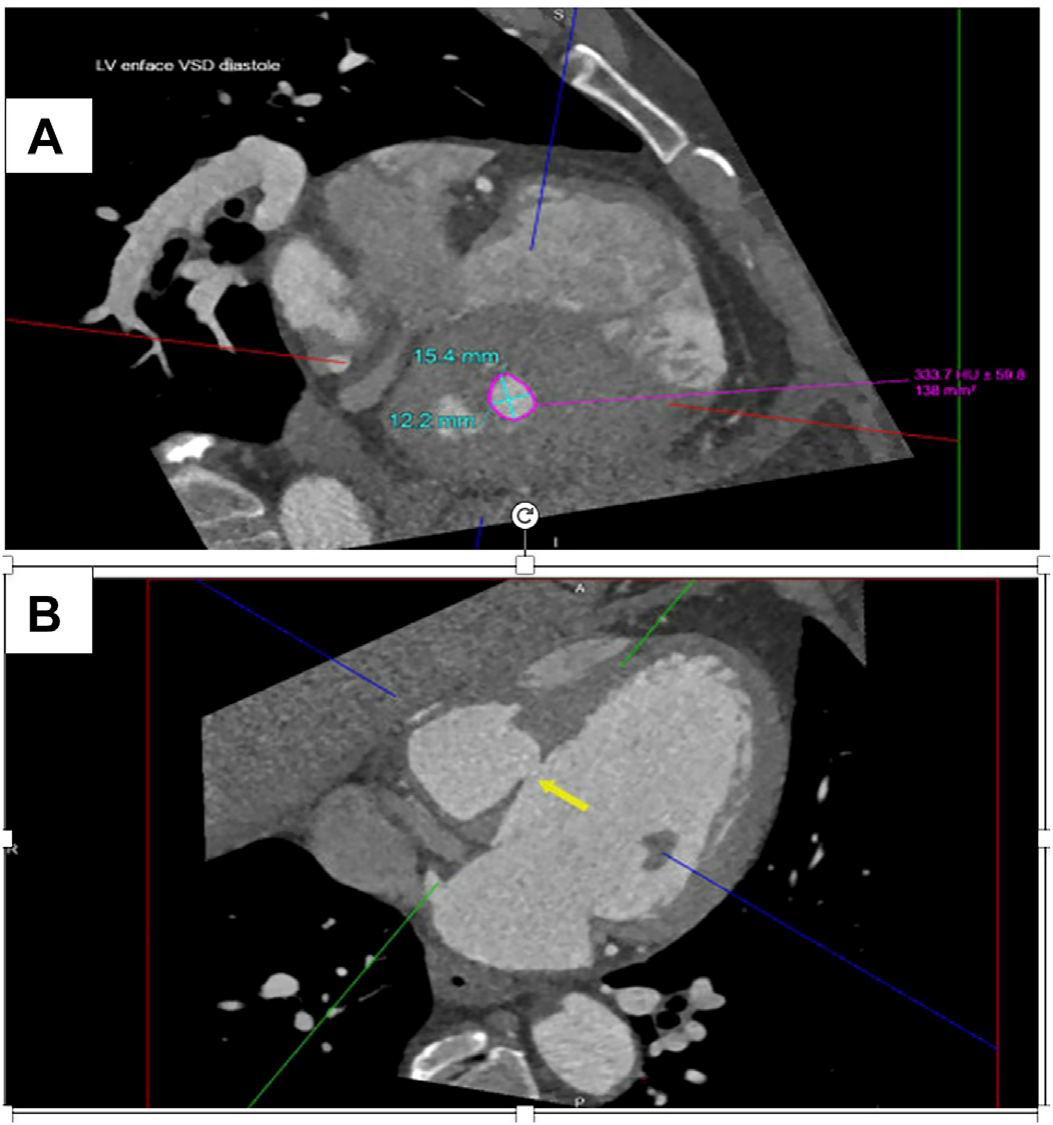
Computed tomography image showing postinfarction ventricular septal defect. (A) shows the Ventricular Septal Defect (VSD) as seen enface from the left ventricle (LV) and the dimensions of the VSD in diastole (blue X). (B) shows the location of the defect along the interventricular septum and shunt across the septum (yellow arrow).
